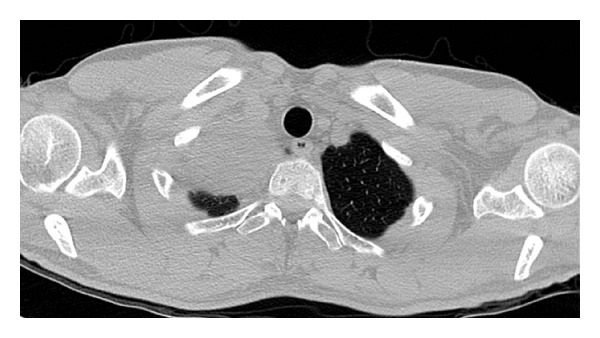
CT scan (CT) (axial view) showing a well-circumscribed, encapsulated ovoid mass, measuring approximately 5 cm, located in proximity to the right thoracic outlet.
